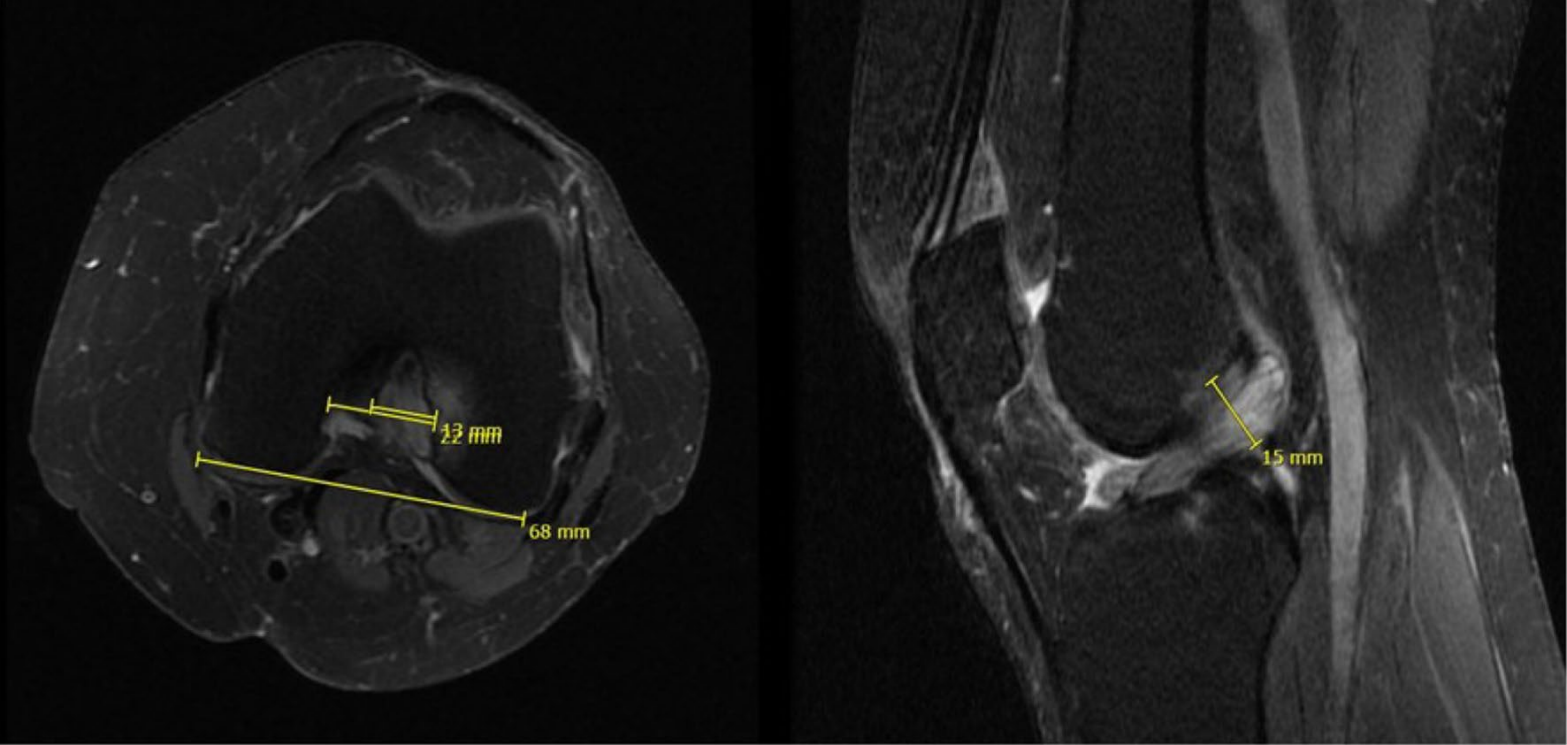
Measurement in the MRI of the MDACL: ratio of the ACL and intercondylar notch width on the axial view and ACL thickness on the sagittal view
